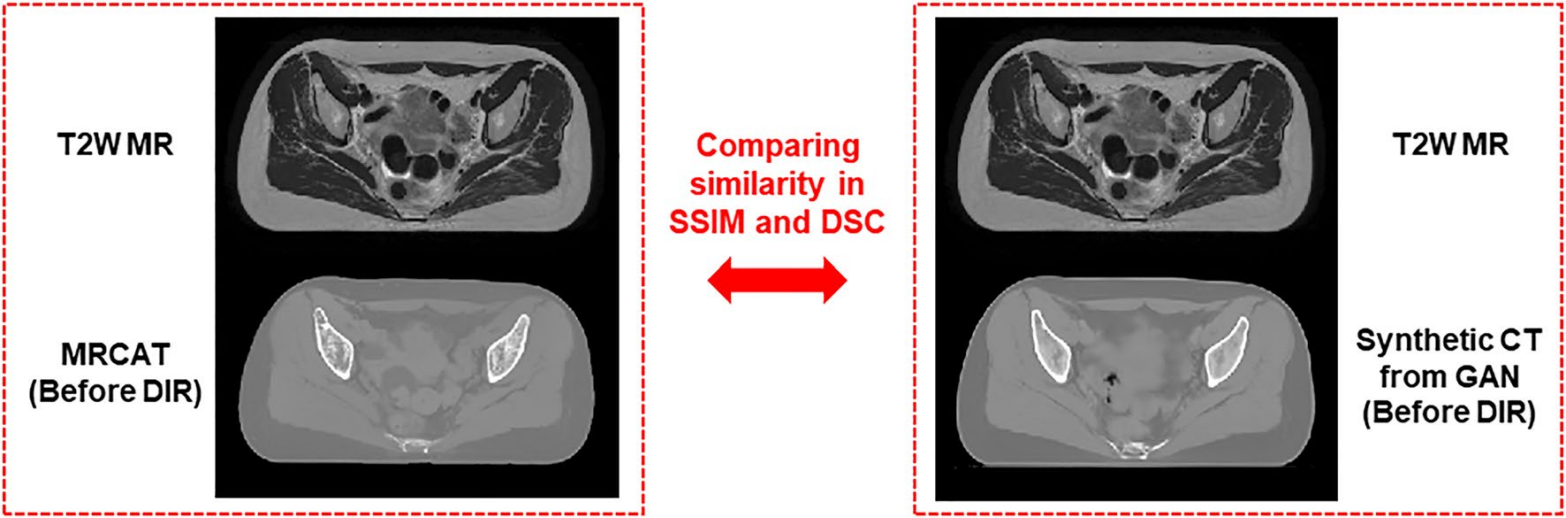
Comparing image similarity between (1) T2W MR and MRCAT (with no DIR), and (2) T2W MR and GAN-based synthetic CT images (with no DIR) to identify that the deformable image registration is independent of superiority of a specific approach against the other in generating the synthetic CT images.
